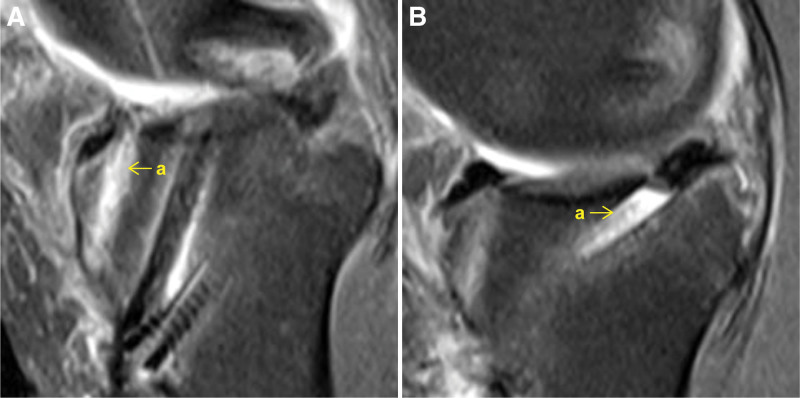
Postoperative sagittal magnetic resonance images. (A) a, anterior intratunnel tendon autograft. (B) a, posterior intratunnel tendon autograft.
